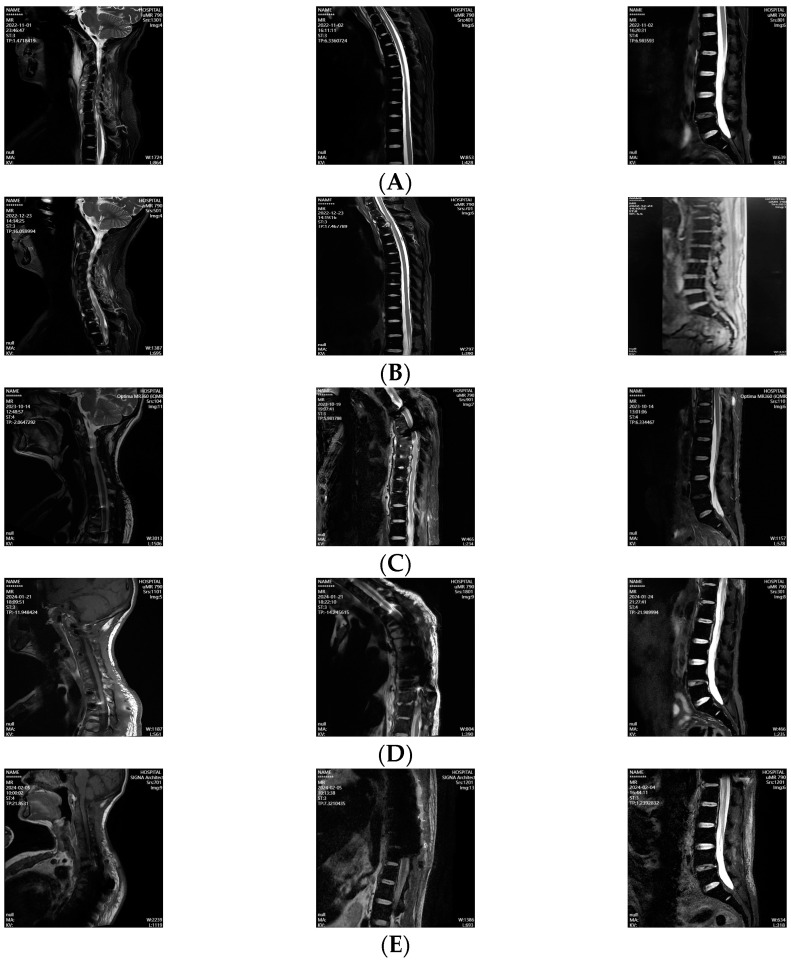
MRI scan of cervical, thoracic, spine, and lumbar spine. (A) Pre-first surgery (November 2022). (B) Pre-second surgery (December 2022). (C) Pre-third surgery (October 2023). (D) Pre-fourth surgery (January 2024). (E) Follow-up imaging (February 2024).
